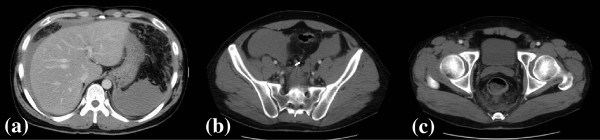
Follow-up contrast-enhanced abdominal computed tomography taken on hospitalization day eight. (a) Decrease in the volume of hemorrhagic ascites (b) An absence of extravasation (c) Increased contrast medium uptake by the rectal walls.
